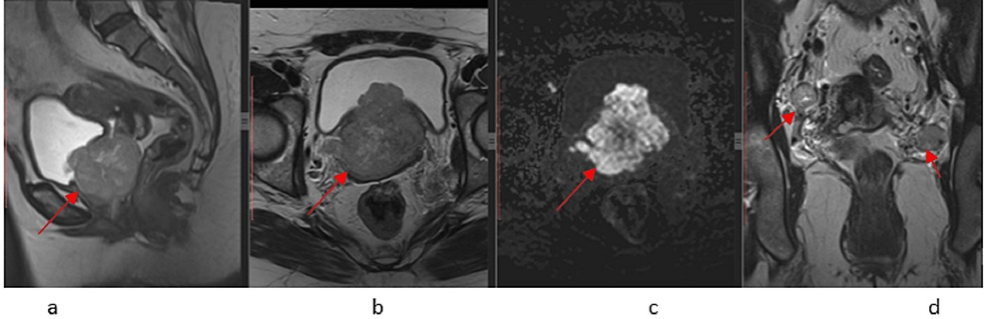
Initial MRI T2 sagittal, axial, and coronal views demonstrate a heterogeneous lobulated mass in the upper two-thirds of the vagina, involving the lower half of the cervix and anteriorly infiltrating into the urinary bladder (1a and 1b), bilateral pelvic side wall lymphadenopathy on coronal T2W1 (1d), and showing restricted diffusion on DWI image (1c) DWI: diffusion-weighted imaging
